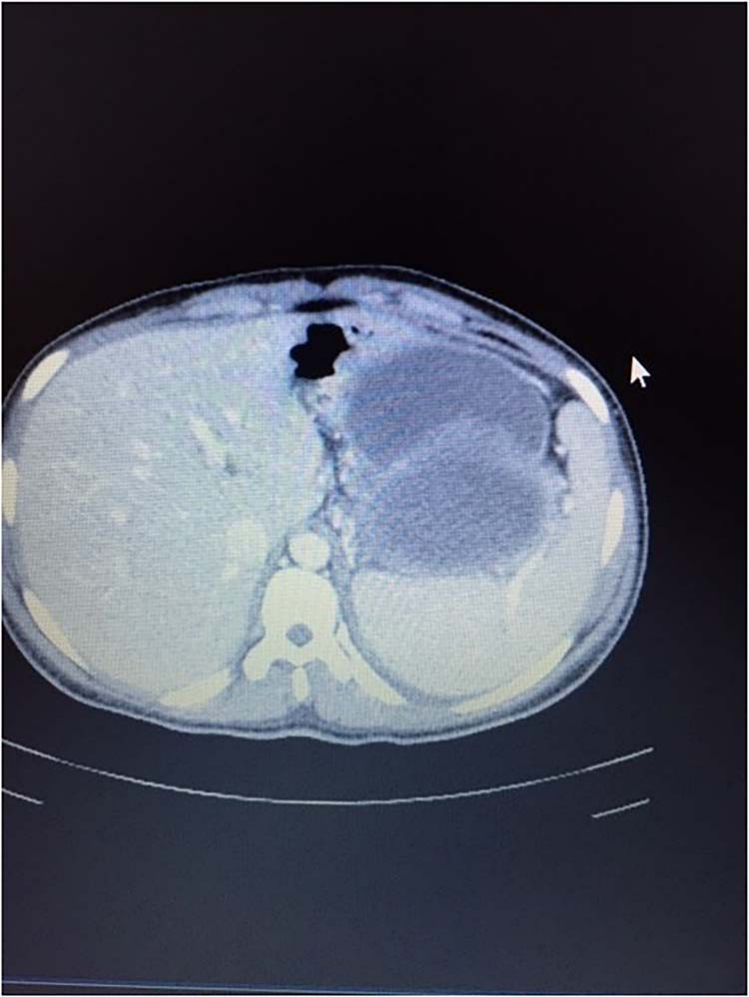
CT scan showing normal liver and enlarged spleen in close proximity with the mass.
